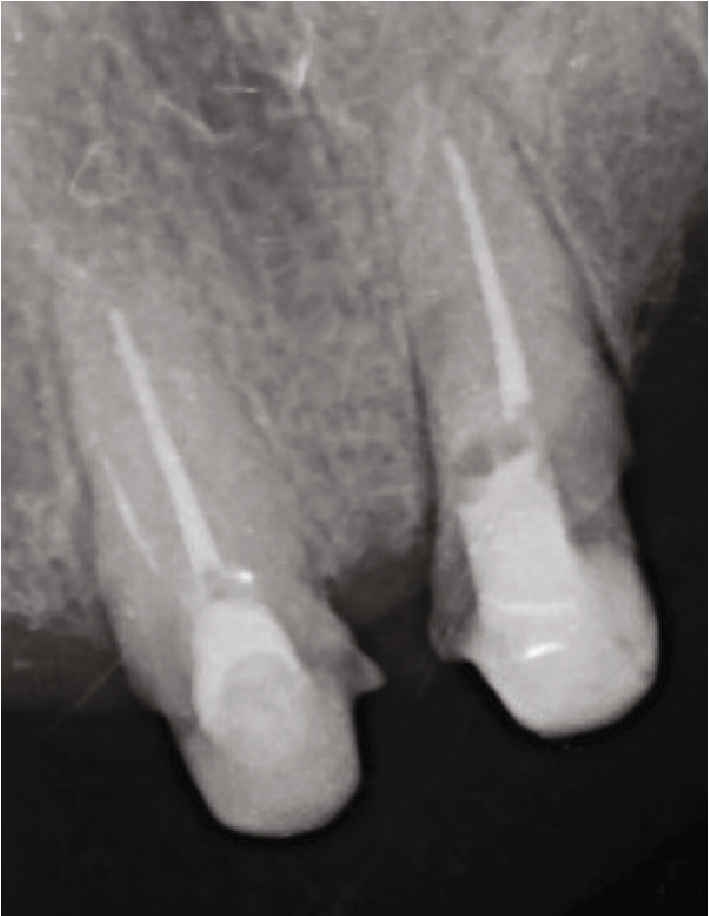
Twelve-month radiographic follow-up.
